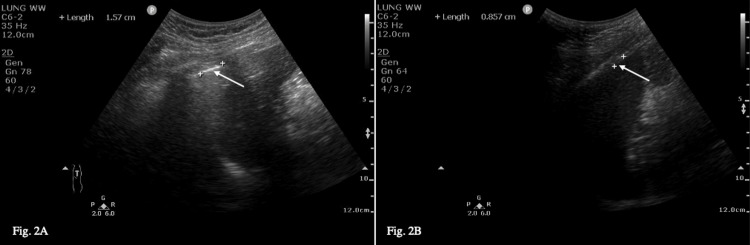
Pre-block (A) and post-block (B) diaphragm ultrasound for case 2.
